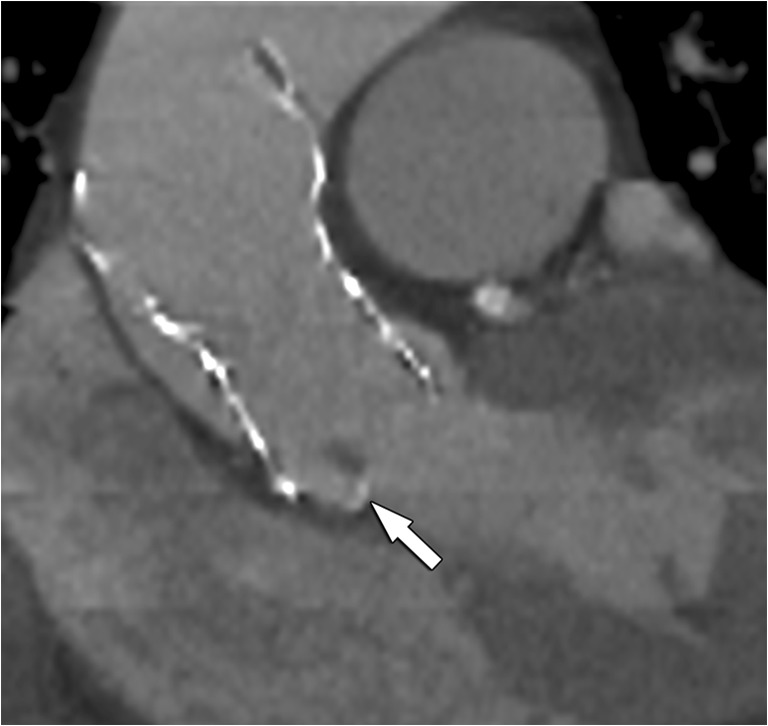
Incorrect positioned THV, which is tilted and does not fully extend into the aortic annulus. As such, parts of the native right aortic valve leaflet is protruding into the inflow part of this self-expandable THV (arrow), causing a residual valve gradient on Doppler echocardiography. CT is very useful in detecting the cause of THV dysfunction in cases where Doppler echocardiography does not provide an answer. In this case, function was improved after balloon dilatation of the inflow part of the THV, further crushing the remaining valve leaflets against the adjacent aortic wall
